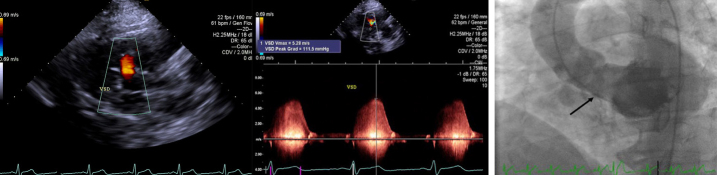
(top left) Transthoracic echocardiogram (TTE) demonstrates muscular ventricular septal defect (VSD) in the parasternal long axis view, (top right) continuous wave Doppler probing demonstrates VSD in TTE, (bottom middle) left ventriculogram demonstrates a small muscular VSD in the left anterior oblique projection.
